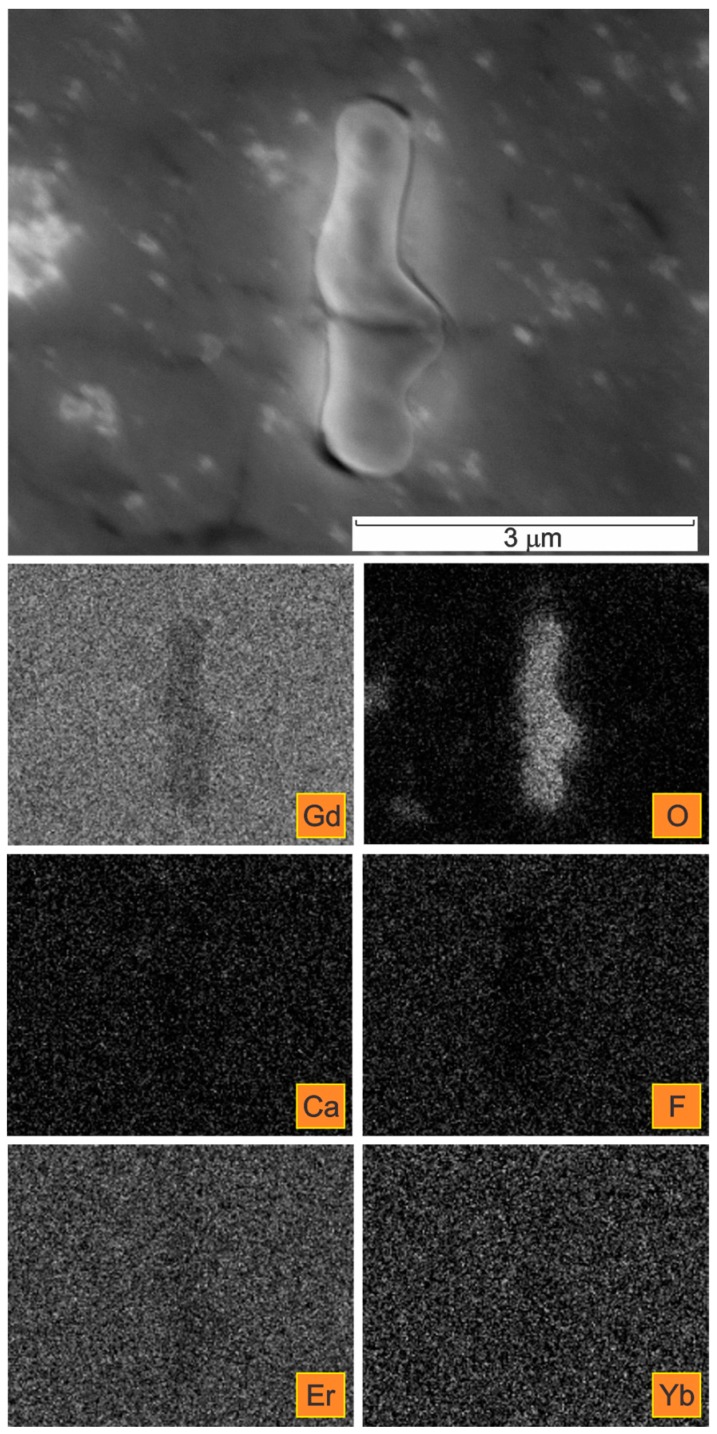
SE image of an oxidized inclusion in a Gd cylinder in the extrusion direction with the corresponding X-ray elemental mappings.
